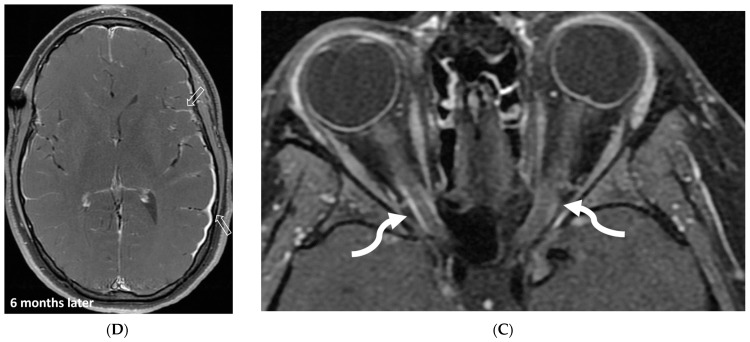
Axial FLAIR (A), Axial T2 orbits (B), Axial T1 orbits post contrast (C) and axial T1 post contrast (D): 13-year-old female with headache and blurred vision. Abnormal FLAIR hyperintensity involving the left parieto-occipital periventricular white matter (arrow), and bilateral cerebellar hemispheres. Bilateral papilledema (black arrow) and edematous left optic nerve (dashed arrow). Peripheral optic nerves/optic sheath enhancement in the posterior aspect (curved arrows). Demyelination, infectious and metastatic processes were considered. MRI brain 6 months later with persistent symptoms demonstrates patchy and asymmetric pachymeningeal and leptomeningeal enhancement (open arrows). Pathology: Diffuse Leptomeningeal Glioneuronal Tumor.
